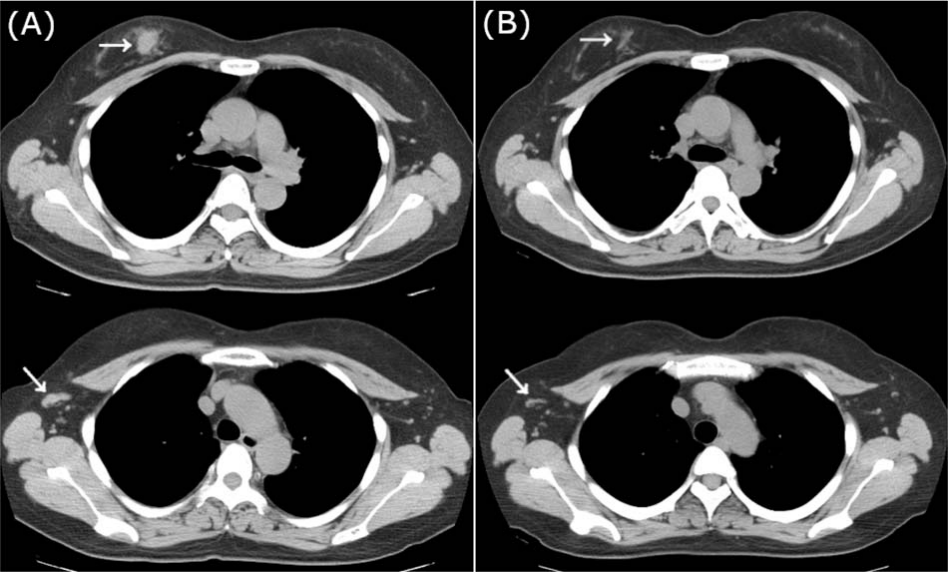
CT images of right breast tumor before and after treatment. (A) Chest CT displaying nodules in the right breast (top, arrowhead) and lymph node swelling in the right axilla (bottom, arrowhead); (B) after administration of NAC, the primary tumor (top, arrowhead) and axillary lymph nodes (bottom, arrowhead) displayed a clear reduction in size.
